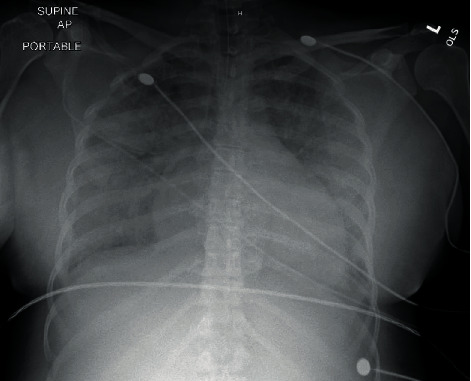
Chest X-ray demonstrating mild cardiomegaly with moderate pulmonary edema after cardiopulmonary arrest.
